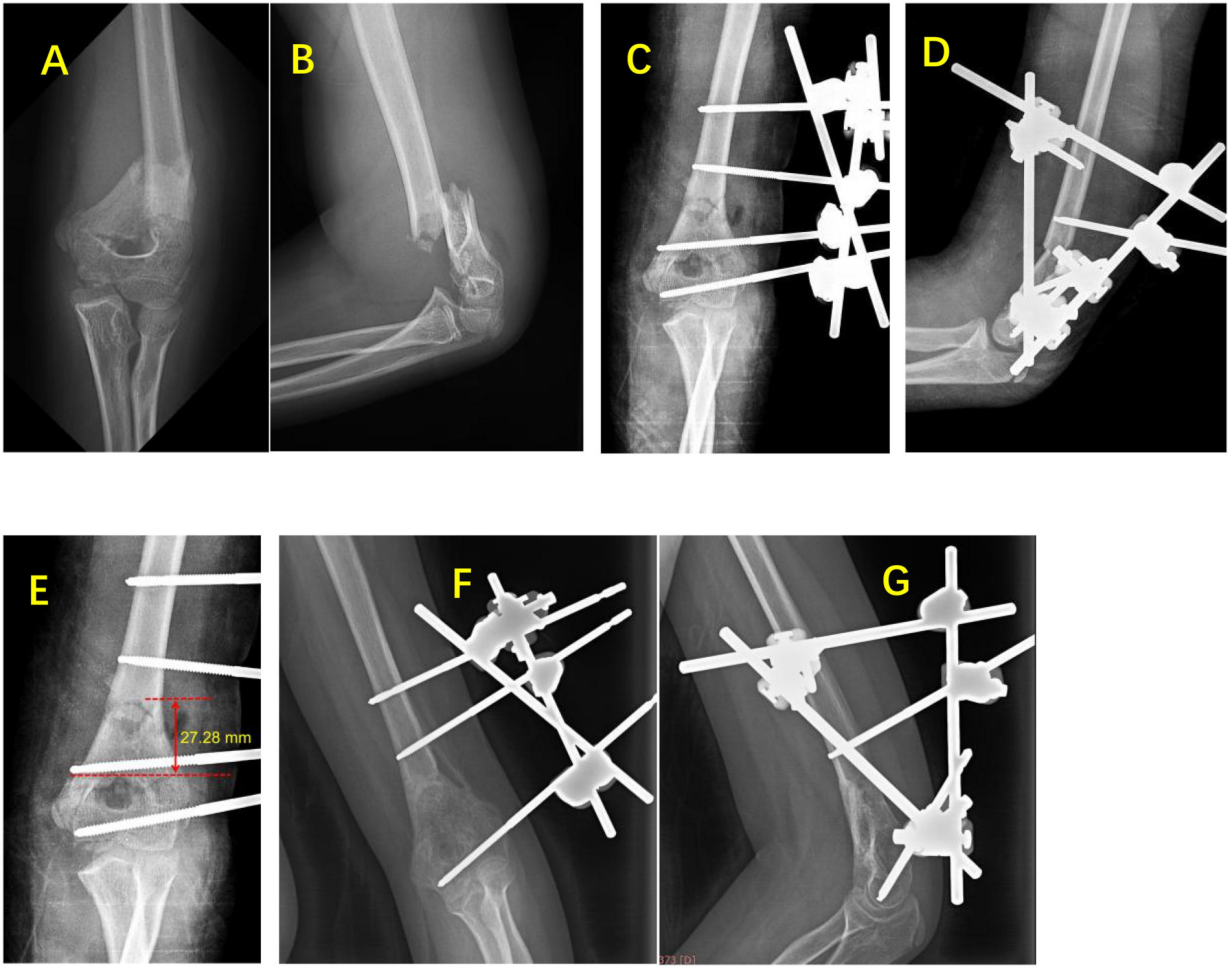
Representative case of triangular configuration external fixation. A 9-year-4-month-old girl with left distal humeral MDJ fracture (transverse type). (A,B) Preoperative radiographs. (C,D) Intraoperative radiographs after triangular external fixation (no K-wires). (E) Fracture line height: 27.28 mm. (F,G) 5-month follow-up showing complete union; external fixator removed (one distal half-pin removed at 3 months).
